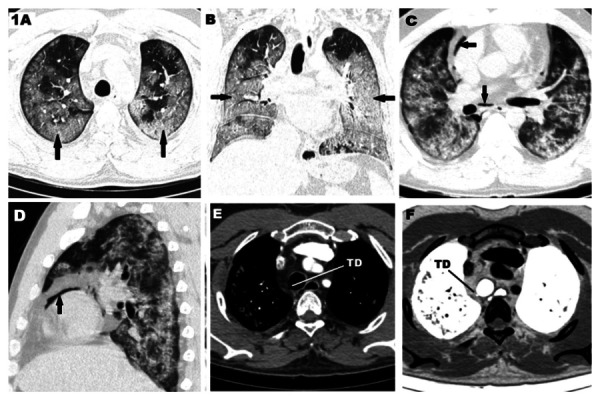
CT scan of a 50-year-old male, RT- PCR positive SARS-CoV-2 patient showing. A. Axial HRCT scan showing multifocal ground glassings in both lung fields typical of covid 19 lesions (arrows). B. Coronal HRCT scan showing extensive involvement in both lungs (arrows). C&D. Axial and sagittal CT scan showing pneumomediastinum (arrows) along with lung lesions aﬅer non-invasive ventilation. E&F. showing the tracheal diverticulum (TD) in axial mediastinal window and axial inverted CT images respectively.
